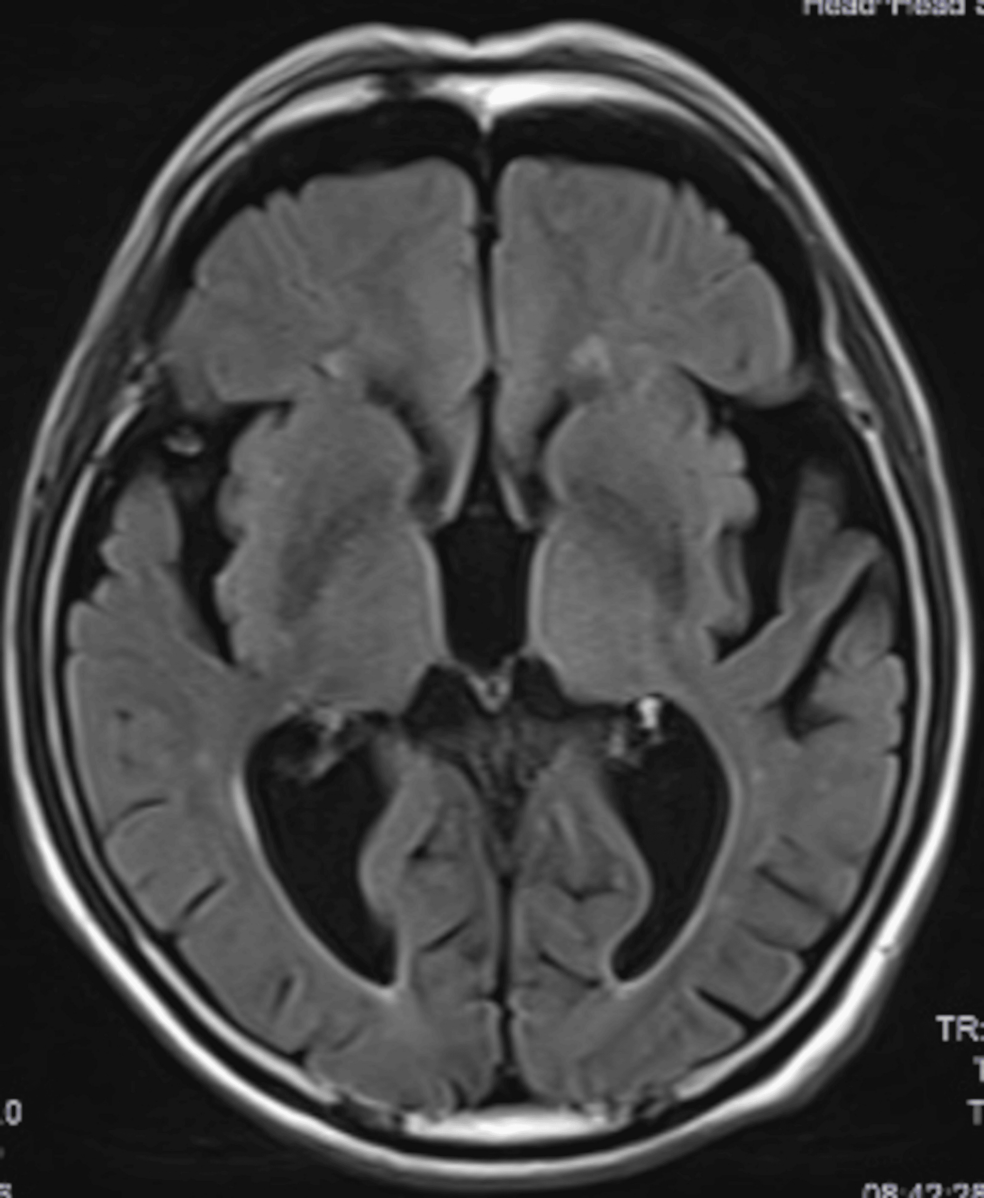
MRI of the brain showing generalized atrophy and an enlargement of the third ventricle MRI: Magnetic resonance imaging
